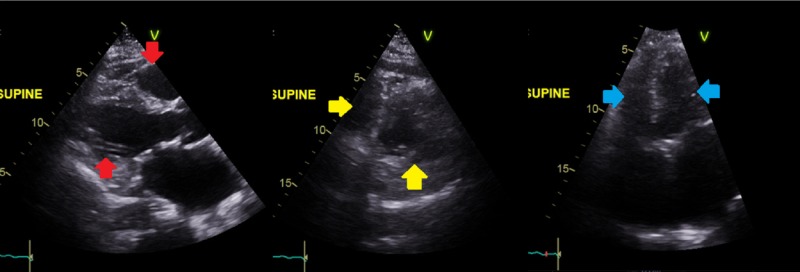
Transthoracic echocardiogram showing normal biventricular size and function in parasternal long axis (red arrows), parasternal short axis (yellow arrows), and apical four chamber (blue arrows) views
